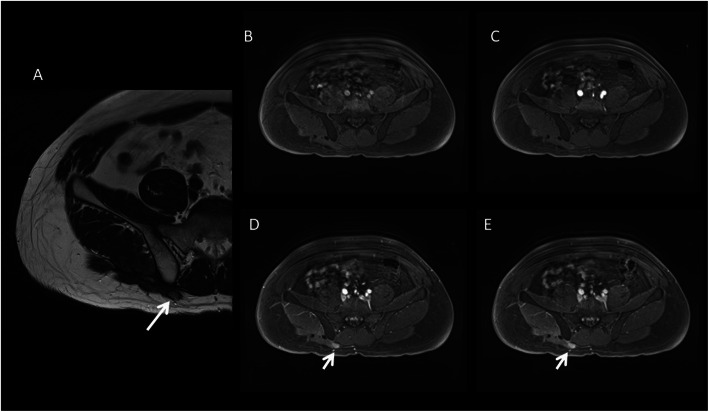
(Same patient of Fig. 3) After surgery, the MRI showed a small nodule (white arrow) characterized by mild hyperintensity on T2wi (a) and by late-progressive enhancement on dynamic T1wi (b T1 before contrast, c arterial phase, d portal phase, e late venous phase)
